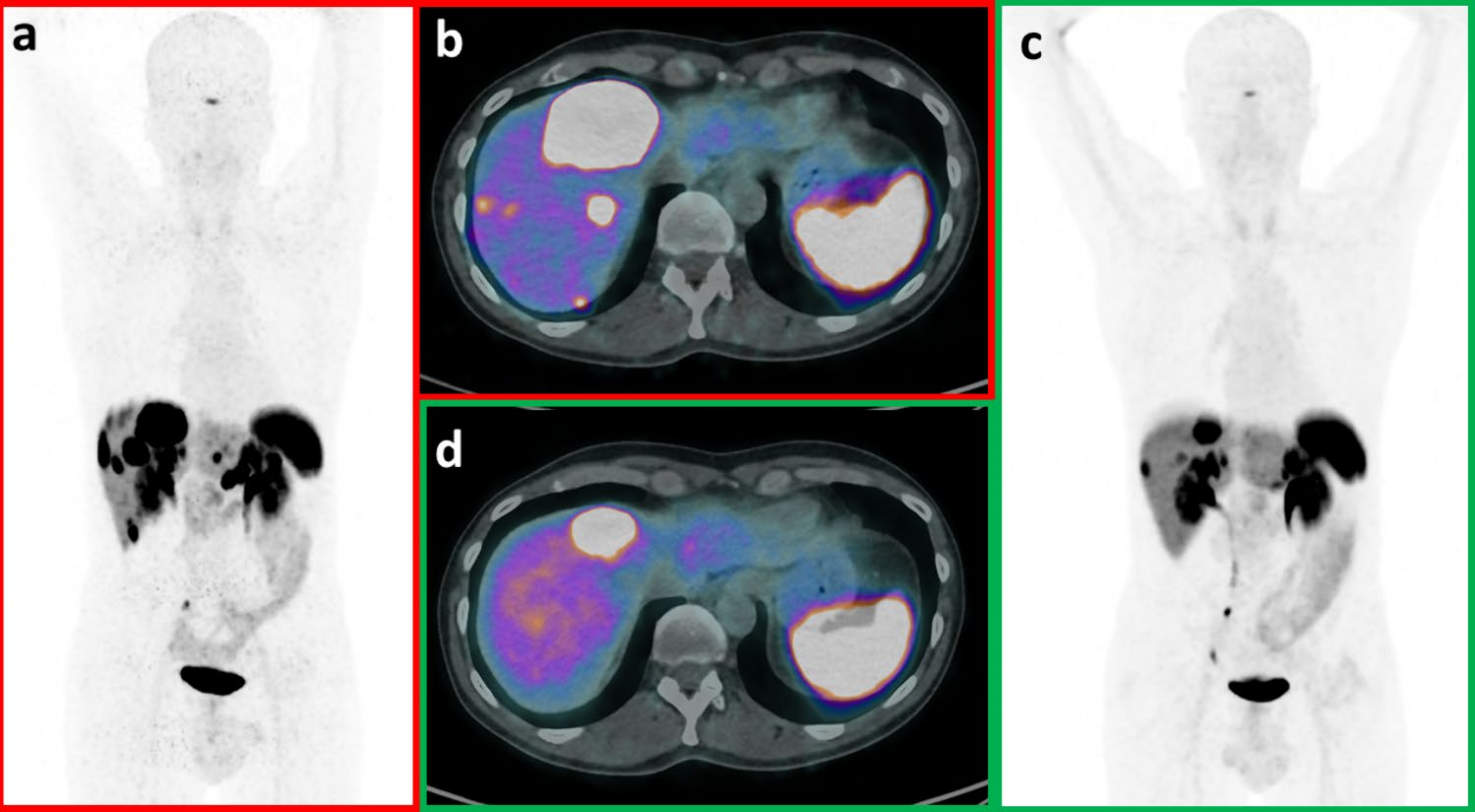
Patient with pancreatic NET G1 (Ki-67, 1.7%) treated with 4 cycles of PRRT with [177Lu]Lu-DOTATATE. Baseline [68 Ga]Ga-DOTANOC PET/CT (a, b) showed high uptake in multiple liver lesions. [68 Ga]Ga-DOTANOC PET/CT after PRRT (c, d) documented reduced lesions’ number and reduced intensity of uptake, in keeping with partial response to PRRT
